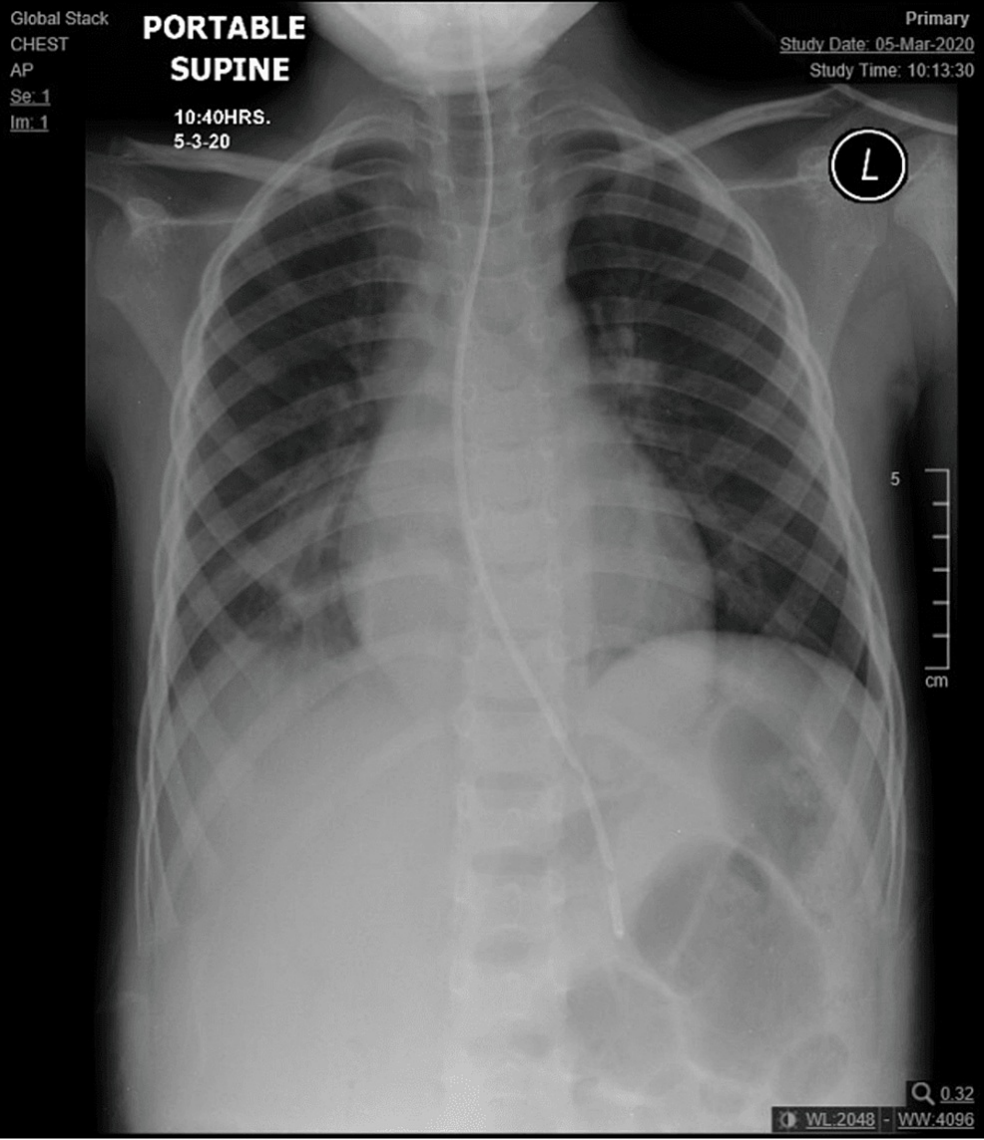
Post-operatively the chest X-ray
